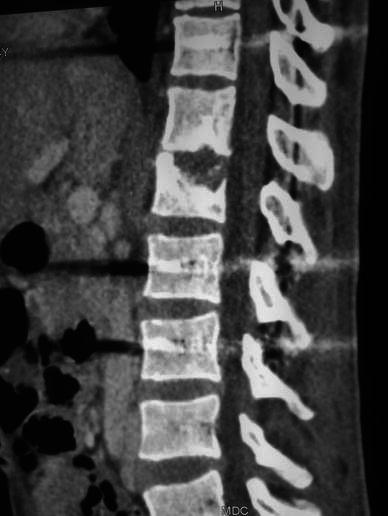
Computed tomography scan 12 months after surgery. Apposition of new bone tissue in T12, improved bone quality of L1, and maintenance of screws positioning
